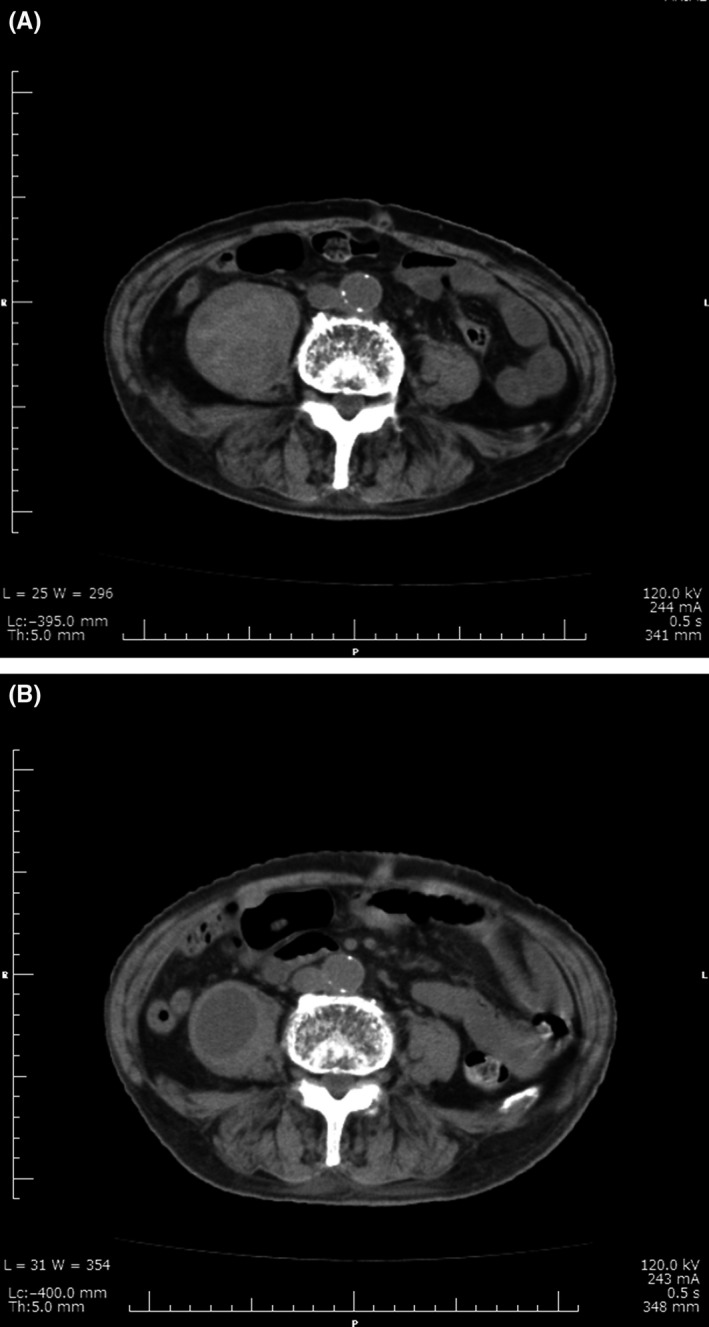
A, Computed tomography (CT) of abdomen and pelvis. CT revealed an approximately 5.4 × 5.8 × 20.0 cm right iliopsoas hematoma. B, Follow‐up CT of abdomen and pelvis. CT on day 76 revealed reduced hematoma
